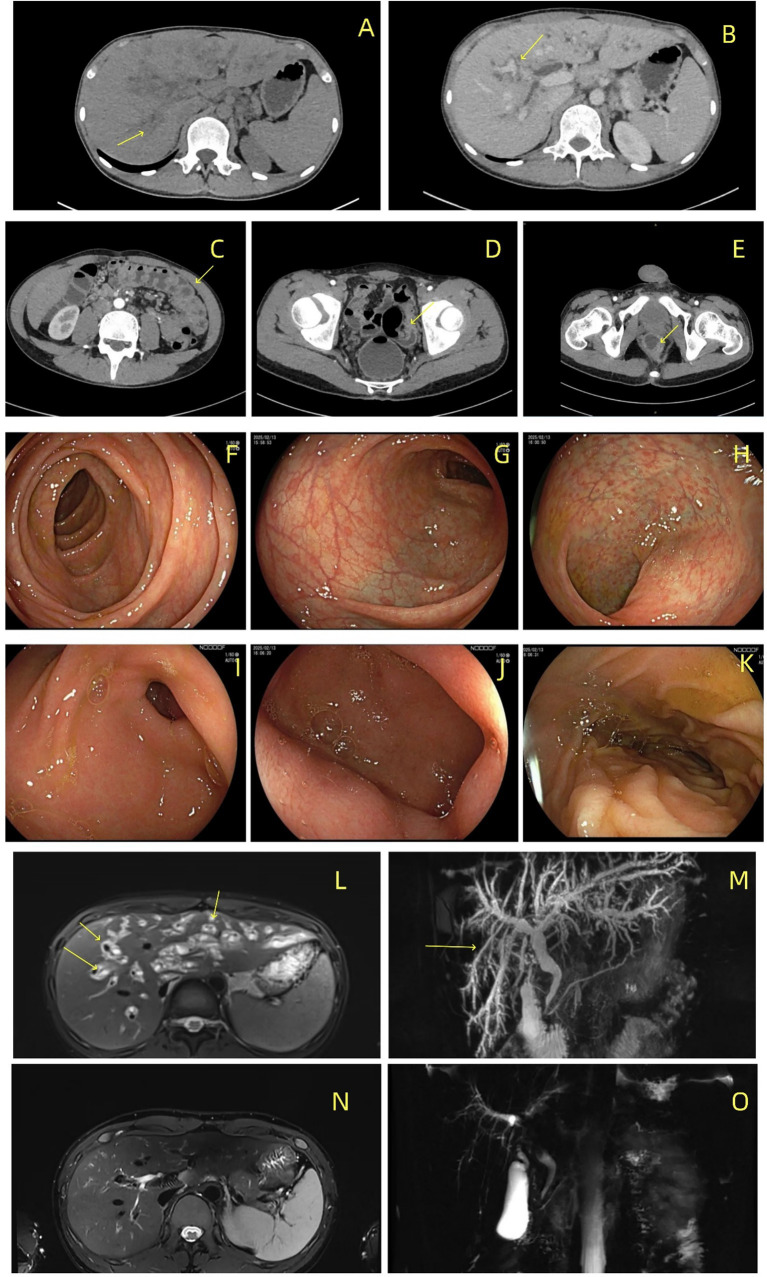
Changes in the bile ducts and the bowel. (A,B) Abdominal CT shows dilation of the intrahepatic and extrahepatic bile ducts, with wall thickening and enhancement of the wall (arrows). (C–E) Contrast-enhanced abdominal CT demonstrating edematous wall thickening of the transverse, sigmoid, and descending colon. (F–H) Colonoscopy revealed no mucosal ulcers or neoplasms (arrows). Only congestion and erosion were observed in the sigmoid colon. (I–K) Gastric antrum, duodenal bulb, and duodenal descending part in gastro-duodenoscopy are normal. (L,M) Magnetic resonance cholangiopancreatography (MRCP) shows diffuse wall thickening of the intrahepatic and extrahepatic bile ducts, accompanied by mild biliary dilation (arrows). No significant stenosis was observed. (N,O) Follow-up MRCP at four months post-antiretroviral therapy initiation demonstrated complete resolution of the biliary abnormalities.
